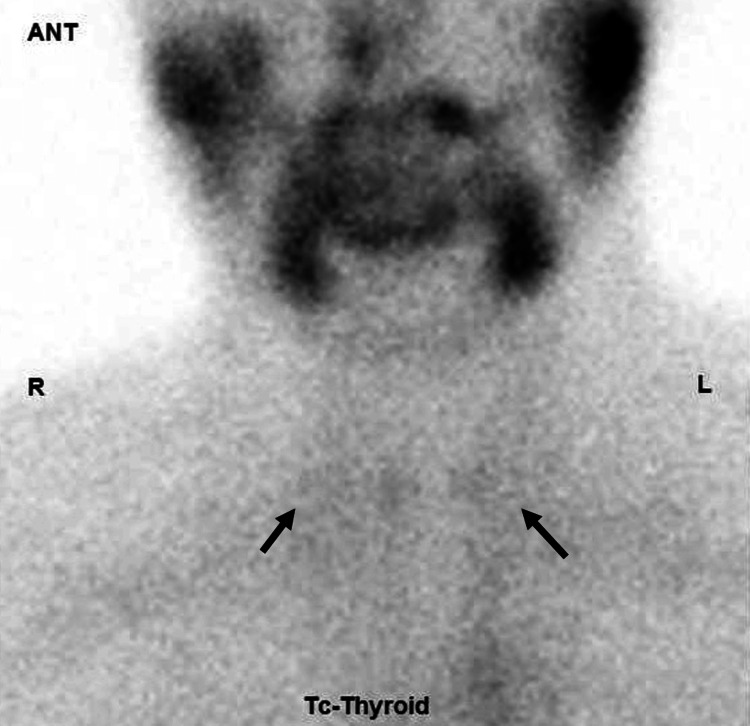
The result of thyroid 99mTcO4 scintigraphy. Thyroid ^99m^TcO_4_ scintigraphy showing almost no uptake in thyroid gland (arrowheads).
